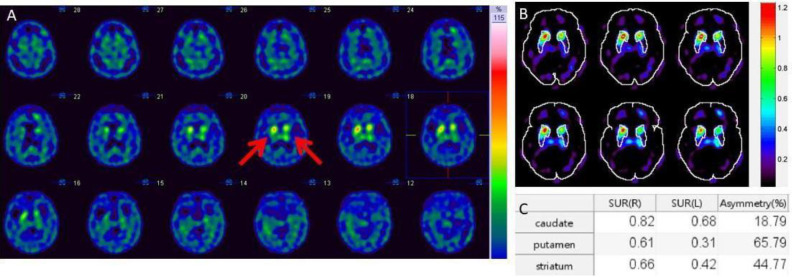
The TRODAT-1 SPECT in Parkinson’s disease with H-Y stage II. A: the TRODAT-1 SPECT showed moderately asymmetrical decreased DAT activity in bilateral putamina, and caudate nuclei. B and C showed a 65.79% asymmetry of putamen uptake. SUR of left putamen is 0.31, while SUR of right putamen is 0.61
